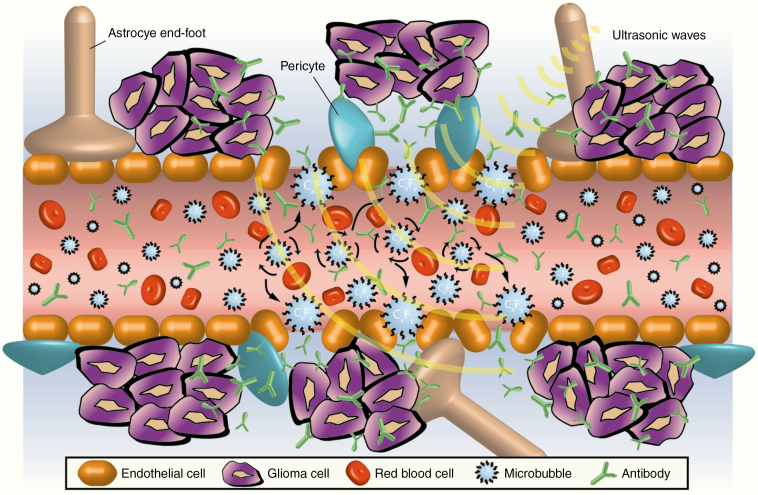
Mechanism of Focused Ultrasound (FUS)-induced BBB disruption. Application of a FUS pulse at the resonance frequency of systemically administered microbubbles causes stable cavitation. This leads to temporary disruption of endothelial tight junctions allowing systemically administered antibodies to penetrate into the brain parenchyma and reach glioma cells.
